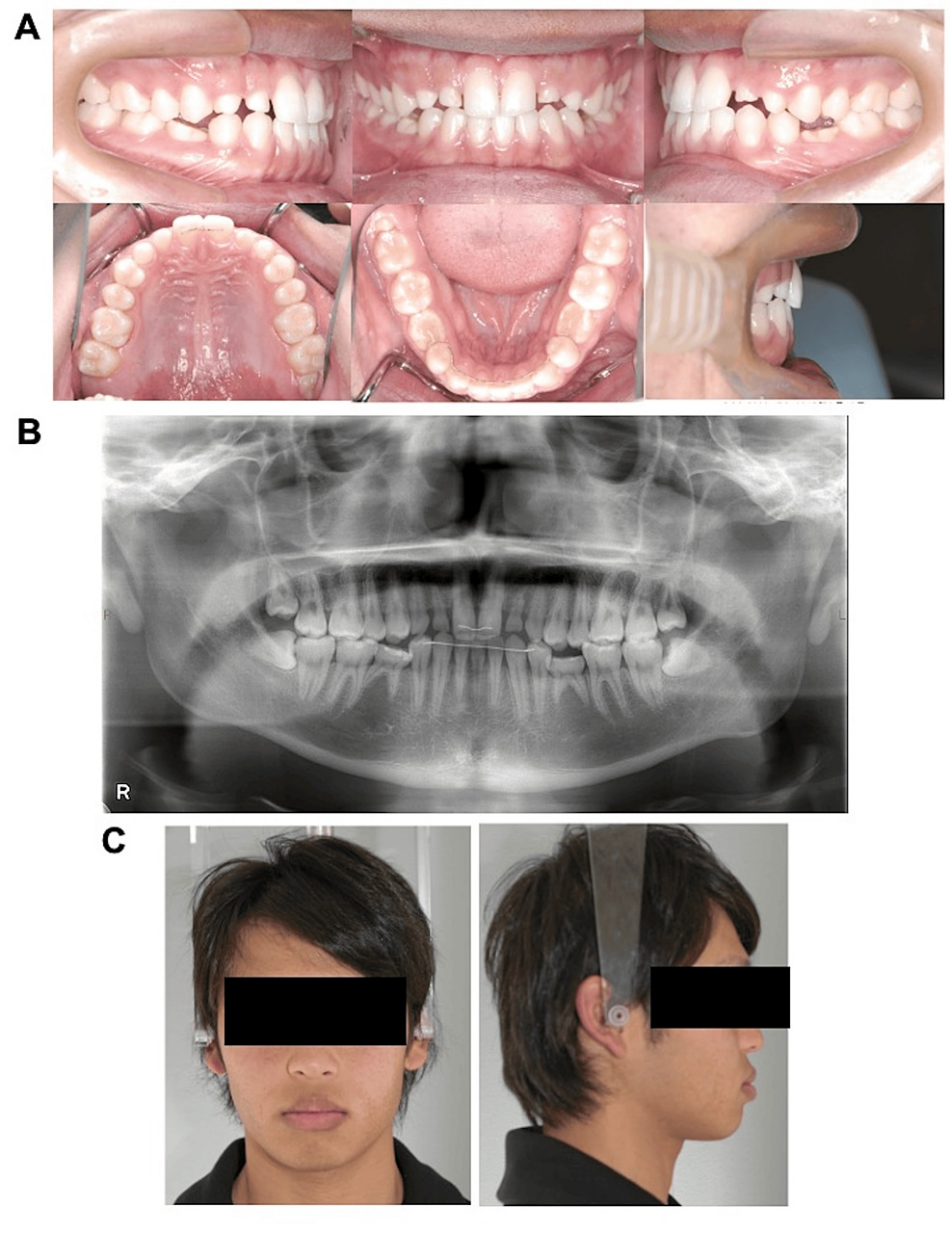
Posttreatment intraoral photograph, facial photograph, and panoramic radiographs A: intraoral photograph obtained at the end of orthodontic treatment; B: panoramic radiography performed at the end of the orthodontic treatment; C: facial photograph obtained at the end of orthodontic treatment.
